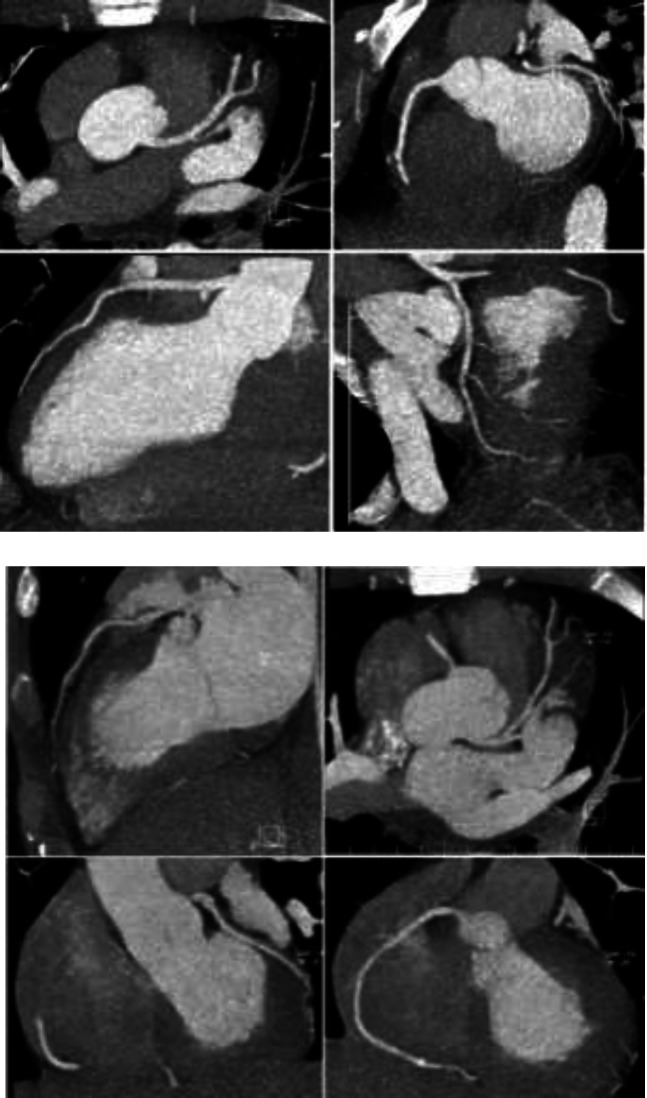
Coronary CT angiography of patient 1 (upper panel four images) and patient 2 (lower panel four images) showing normal appearing coronary arteries
